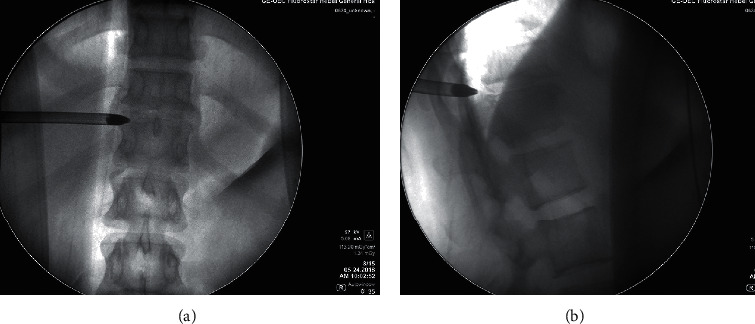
The dilator is located at the lower part of the intervertebral foramen, as can be checked on the lateral X-ray fluoroscopy (a) and anteroposterior X-ray fluoroscopy (b).
